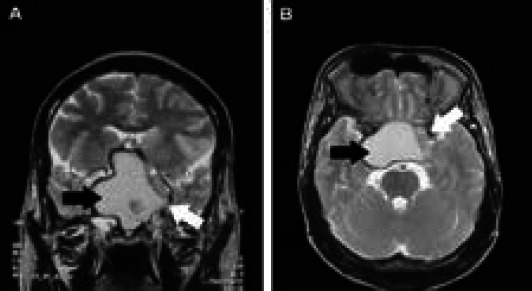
T2-weighted MRI scans of the brain.
